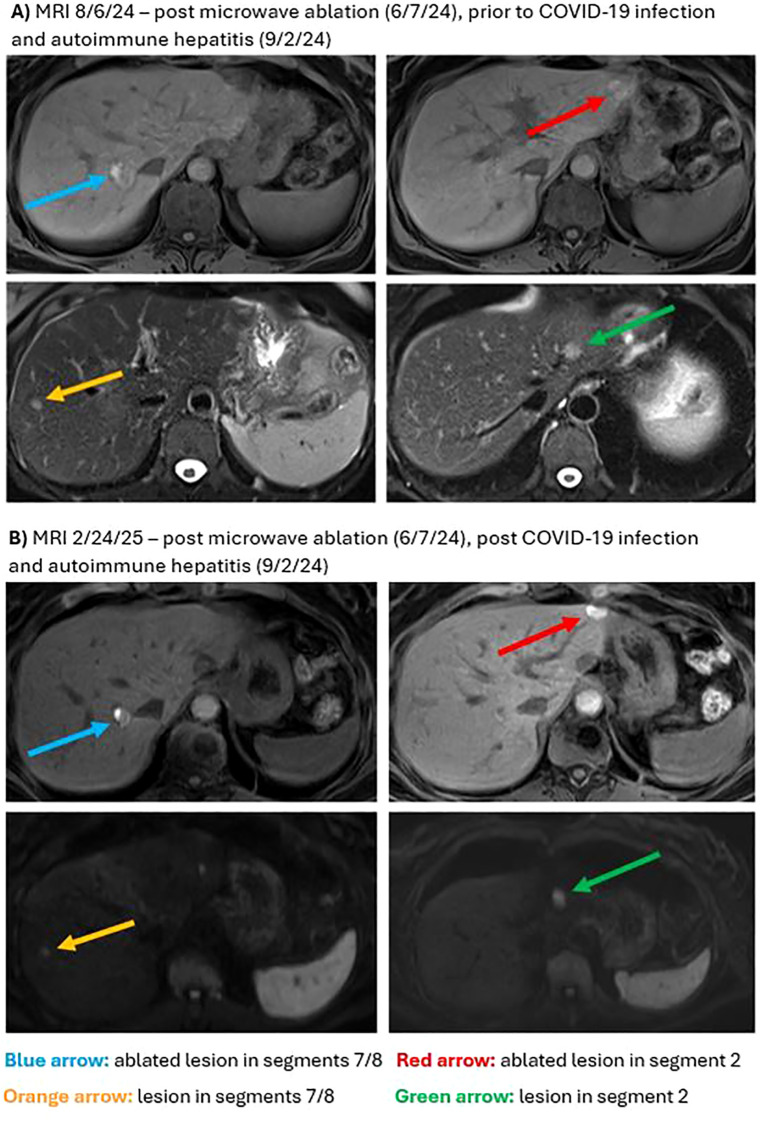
Magnetic resonance imaging (MRI) for hepatic metastases. (A) T1 weighted pre-contrast images from 8/6/24 demonstrate intralesional hyperintensity due to prior ablation in treated lesions in segments 7/8 (blue arrow) and in segment 2 (red arrow). T2 weighted images demonstrate hepatic metastases in segment 2 (green arrow) and in segments 7/8 (orange arrow). (B) Diffusion and T2 weighted MRI images from 2/24/2025 demonstrate unchanged hepatic metastases in segment 2 (green arrow) and in segments 7/8 (orange arrow). Ablated metastases in segments 7/8 (blue arrow) and in segment 2 (red arrow) are less conspicuous on T1 weighted pre-contrast images when compared to 8/6/24.
